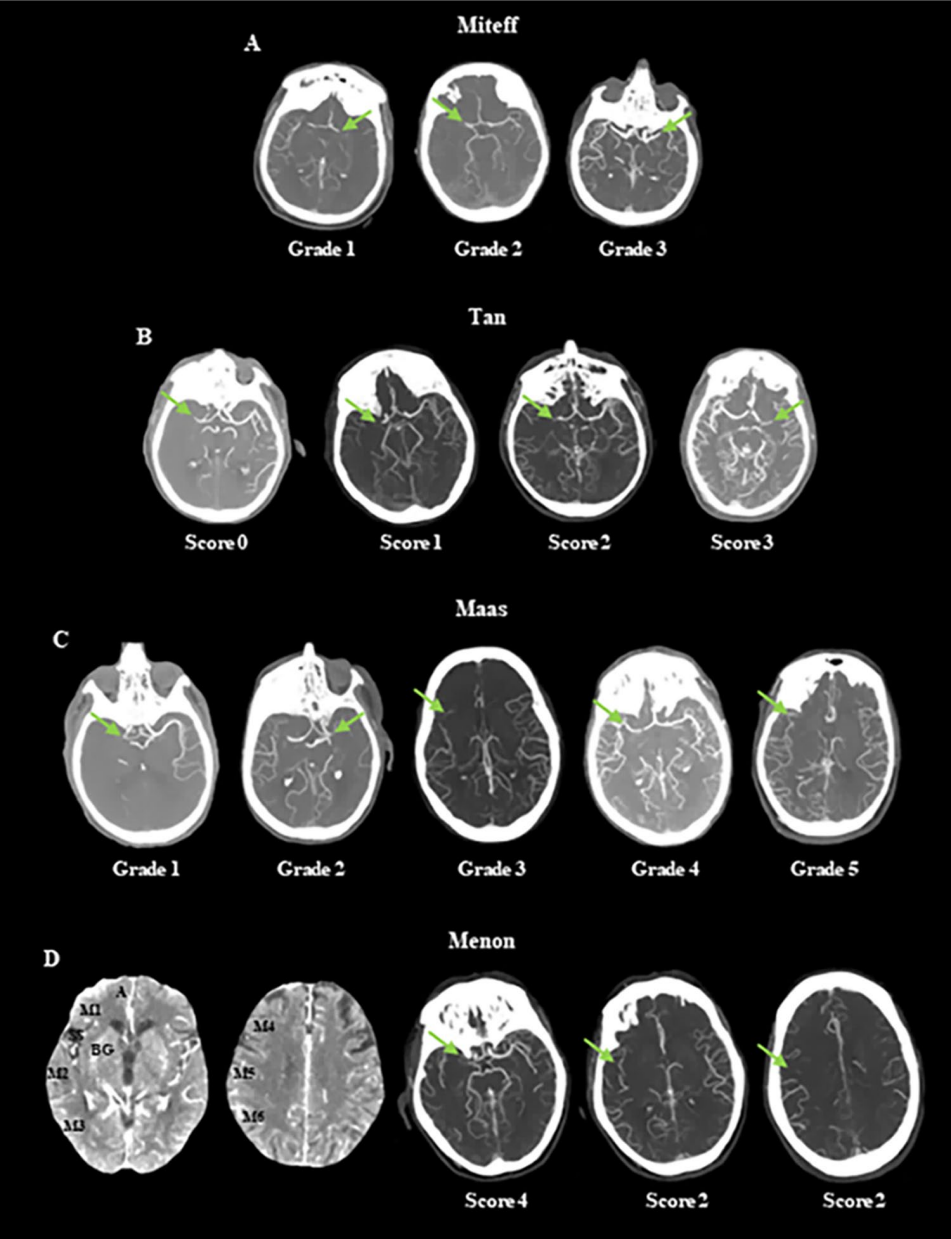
Single-phase CT angiography collateral scores. Green arrows indicate occlusion site or occluded hemisphere. M1, anterior MCA at ganglionic level; M2, lateral MCA at ganglionic level; M3, posterior MCA at ganglionic level; M4, anterior MCA at supraganglionic level; M5, lateral MCA at supraganglionic level; M6, posterior MCA at supraganglionic level; ACA, anterior cerebral artery; BG, basal ganglia
